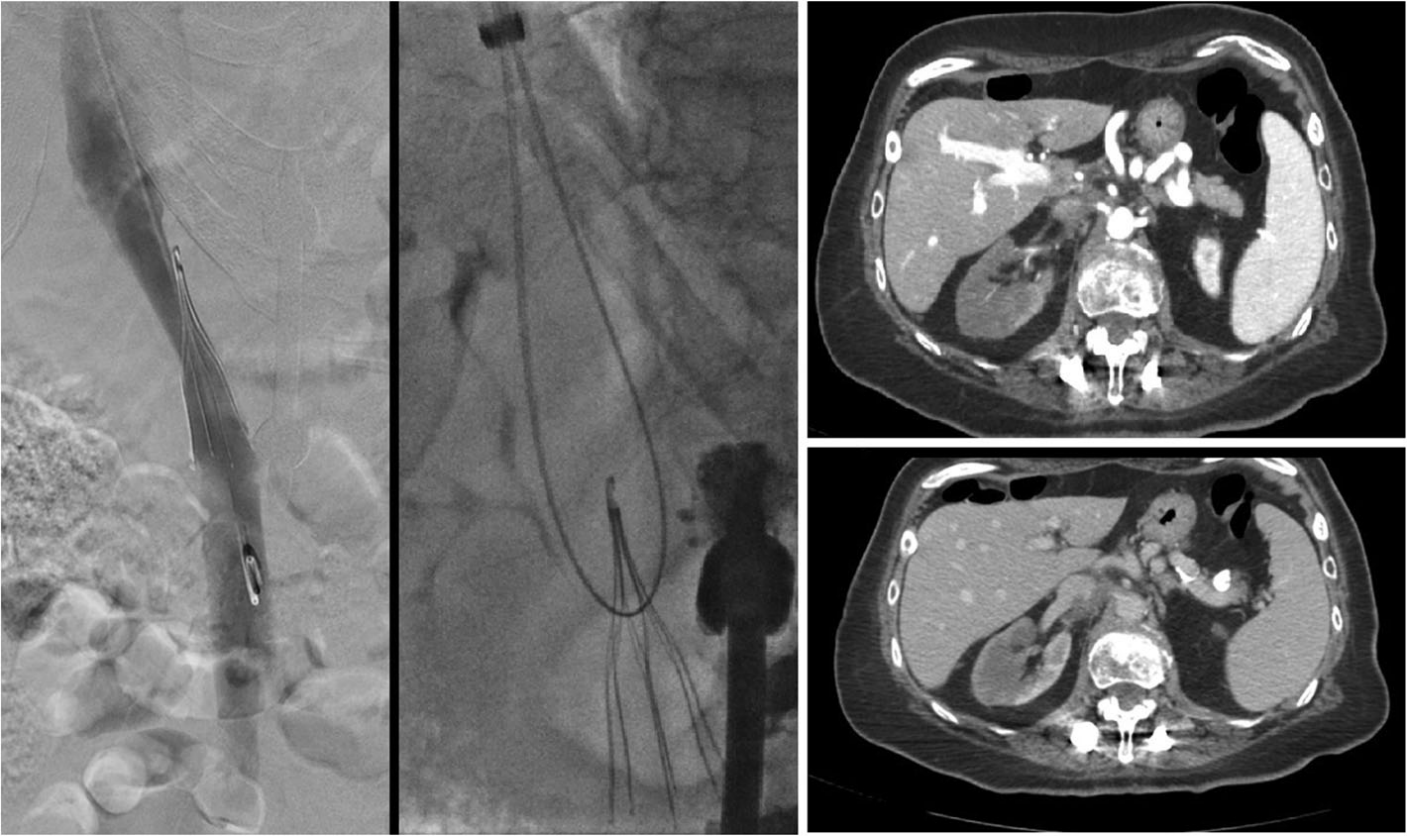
This patient had a pre-operative IVC filter (Argon Option Elite filter; Argon Medical Devices, Plano, TX) placed 3 months prior to attempted removal. Initial cavagram showed the tip to be embedded in the wall of the IVC at the junction of the left renal vein and IVC. Snaring was not possible, so the sling technique was employed. Three days later, the patient had persistent abdominal pain and underwent CT which showed a short segment renal artery occlusion, renal infarction and intramural hematoma of the IVC. Arterial injury after sling technique filter removal has not previously been described in the literature
